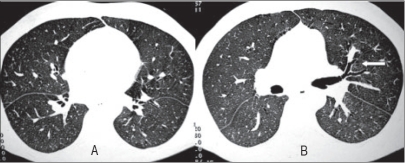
HRCT (axial section) showing presence of ‘dark bronchi’ relative to the surrounding lung parenchyma (arrow)
